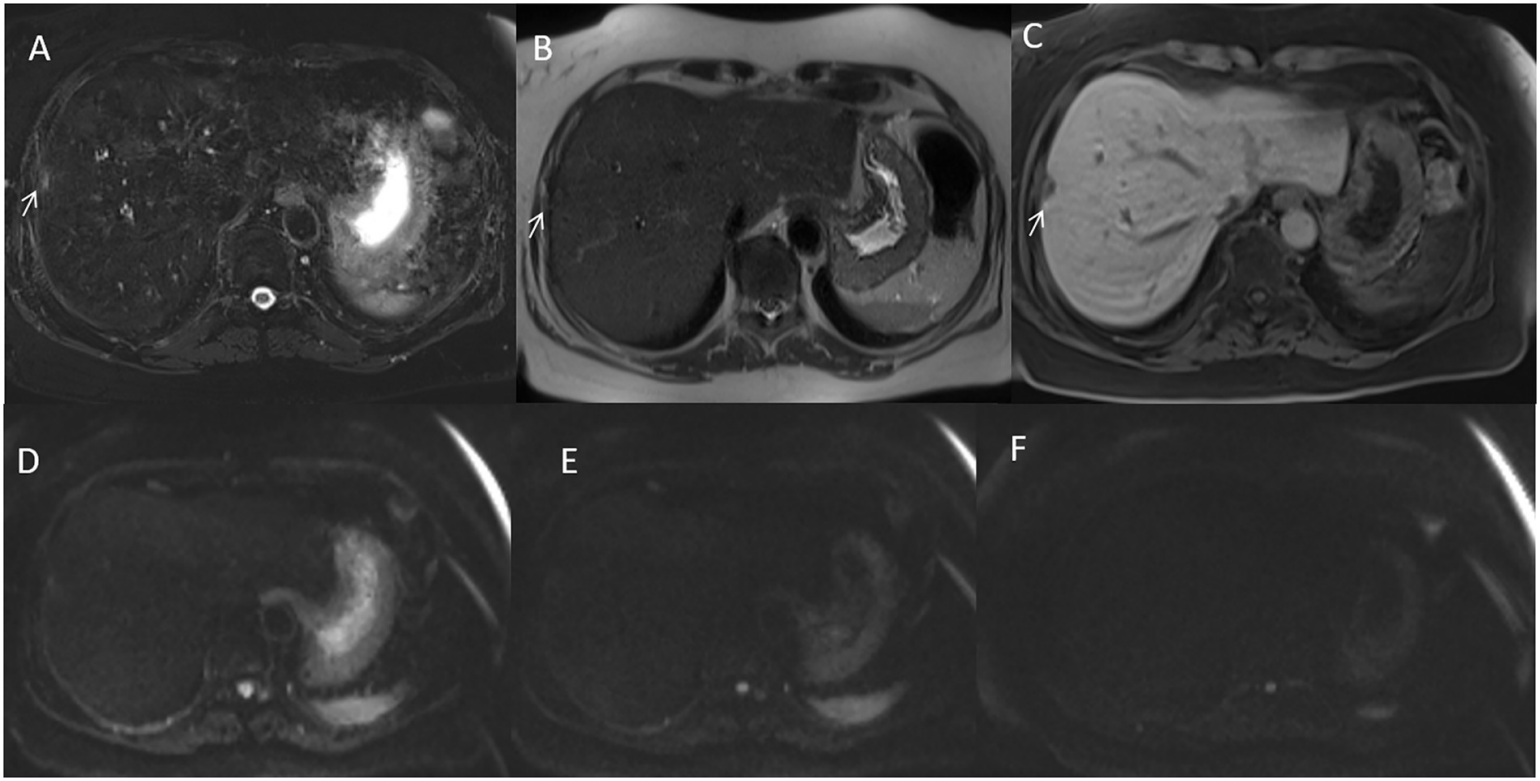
Woman 48 y with left colon cancer. Pre surgical MRI study: In A (SPACE FS T2-W sequence) arrow shows subcapsular lesion. HASTE T2-W sequence (in B) does not detect the lesion, arrow. In EOB phase contrast study the metastasis appear as hypointense lesion without restricted diffusion in DWI sequences (D, E and F).
